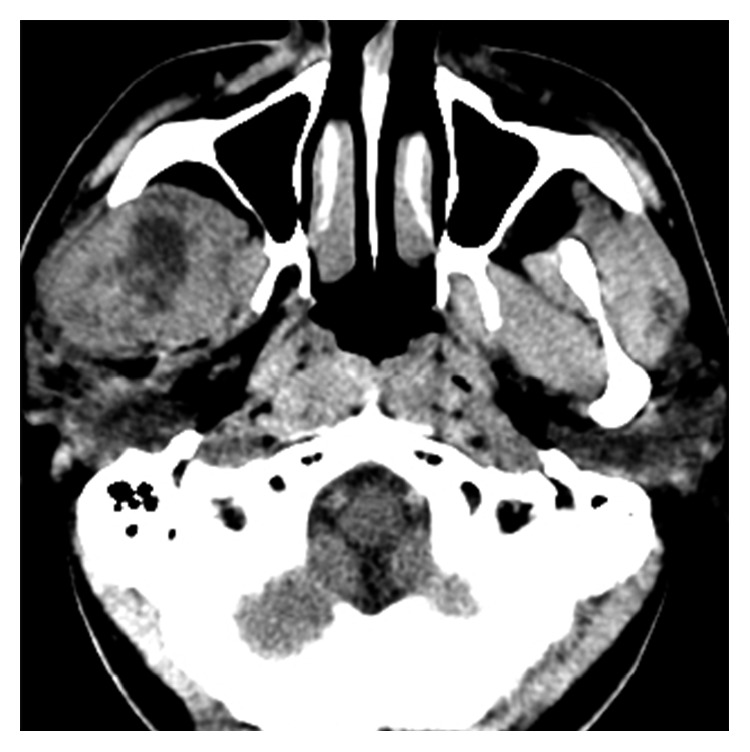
Preoperative CT scan shows the recurrent ameloblastoma in the infratemporal fossa.
